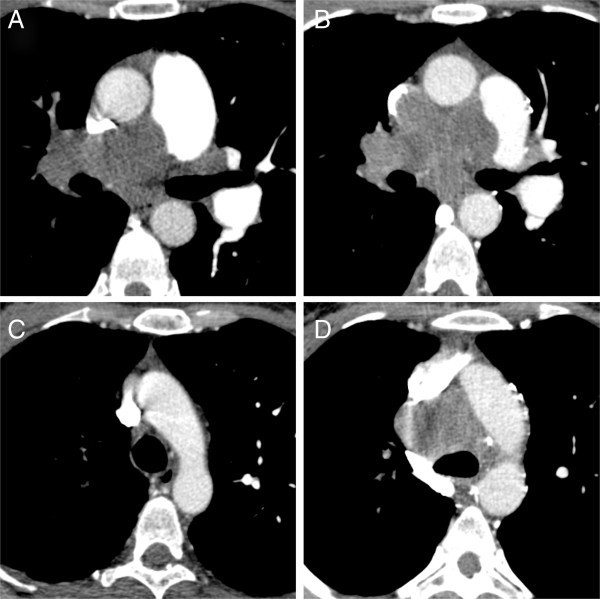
CT pulmonary angiography (CTPA) of a 49-year-old female patient with a myxofibrosarcoma of the right pulmonary artery. Suspicious imaging features were already present in the primary imaging (A, C). However, the patient was treated conservatively for suspected pulmonary embolism. A follow-up exam was acquired three month later and demonstrated massive disease progression with tumor expansion beyond the vessel wall and local lymph node metastases (B, D)
